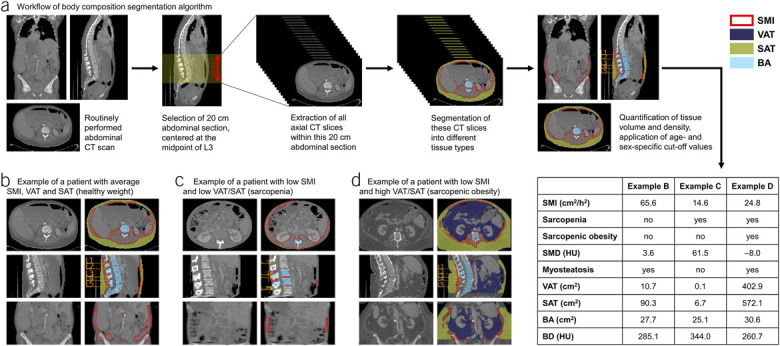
Overview of the body composition segmentation algorithm and representative examples of patients. (a) Body composition was derived from CT scans using a segmentation algorithm that automatically selects a 20 cm section of the abdomen, centered at the midpoint of the third lumbar vertebra (L3). Within this section, all axial CT slices are segmented into different tissue types, after which the quantity and density of these tissue types are measured. Skeletal muscle tissue is outlined in red, visceral adipose tissue is colored purple, subcutaneous adipose tissue is colored green, and bone tissue is colored blue. (b) Example of a patient with average SMI, VAT, and SAT (healthy weight). (c) Example of a patient with low SMI and low VAT and SAT (sarcopenia). (d) Example of a patient with low SMI and high VAT and SAT (sarcopenic obesity). BA, bone area; BD, bone density; CT, computed tomography; SAT, subcutaneous adipose tissue area; SMD, skeletal muscle density; SMI, skeletal muscle index; VAT, visceral adipose tissue area.
